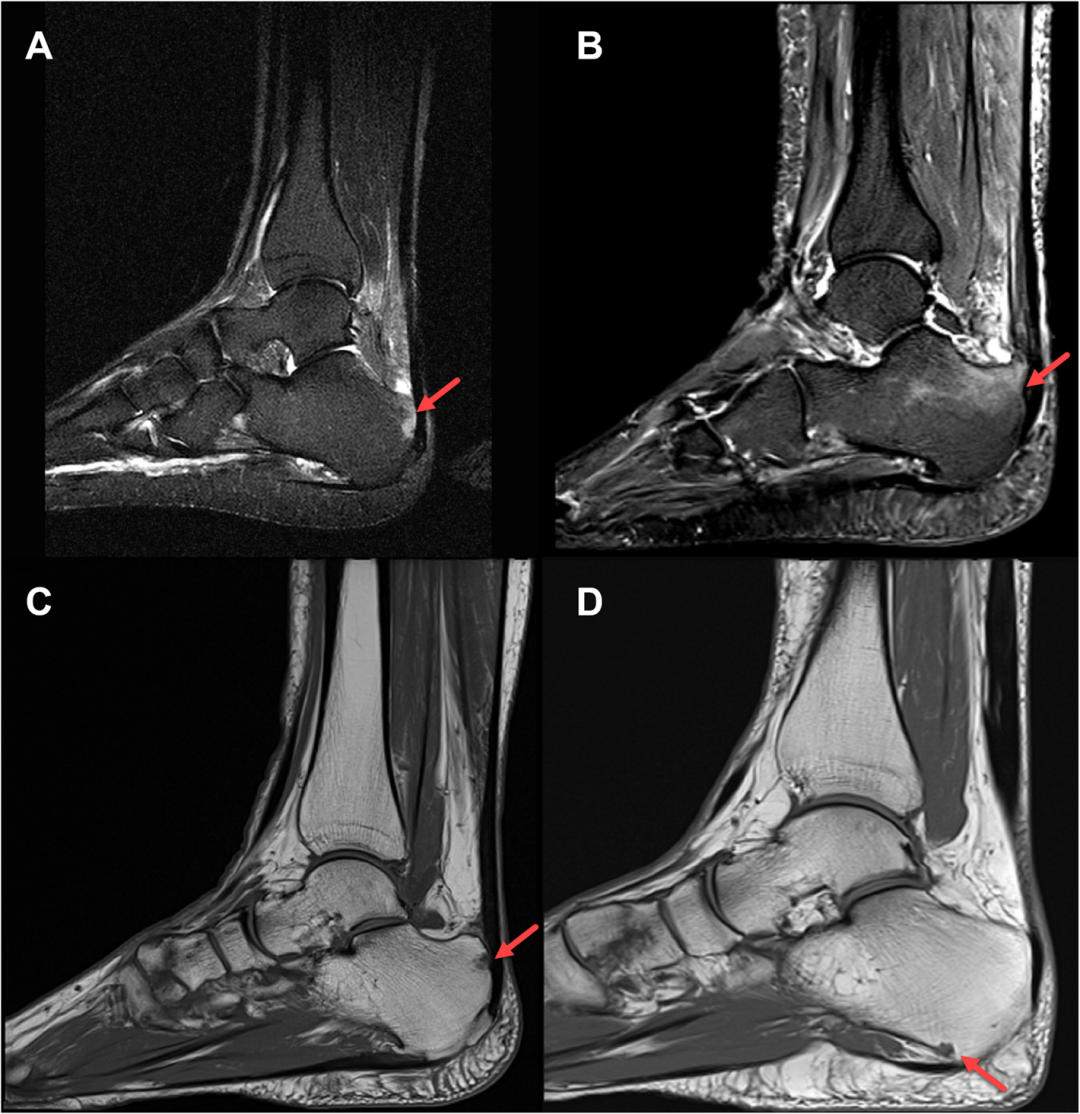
MRIs of bone oedema and bone erosion. a Quantification of bone oedema; 1–33% of bone being oedematous with an oedema length ≤ 0.5 cm. b Quantification of bone oedema; 1–33% of bone being oedematous with an oedema length > 0.5 cm. c Location of bone erosion in the area of the Achilles tendon. d Location of bone erosion in the area of the plantar aponeurosis. MRI, magnetic resonance imaging
